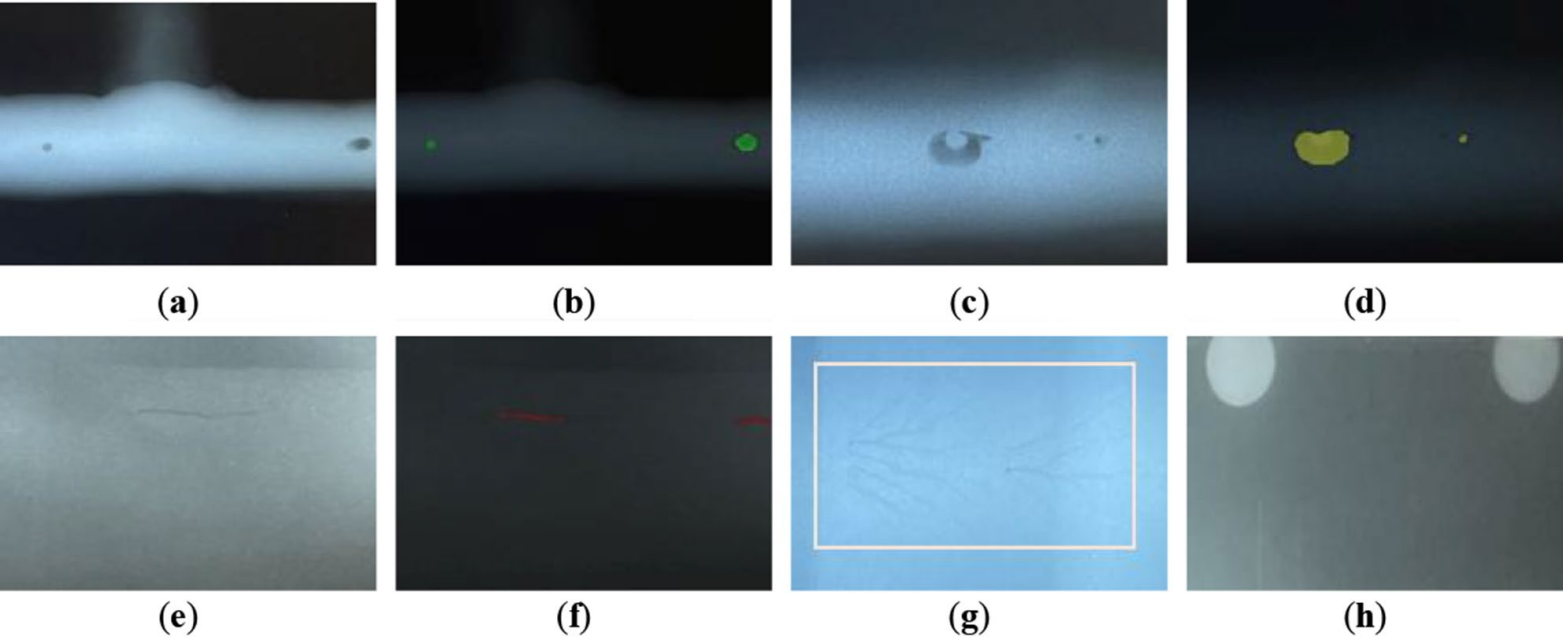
There are results of on-site X-ray image inspection. (a, b): The original picture of the circular defect found in the T-joint of the pressure vessel and the segmentation effect; (c, d): Other defects (pinch bead defects) of the original and segmentation effect; (e, f): The original picture of slight bar and segmentation effect; (g, h): Electrostatic interference and taint interference, correctly treated as background in identification, are not counted in the defect rating.
